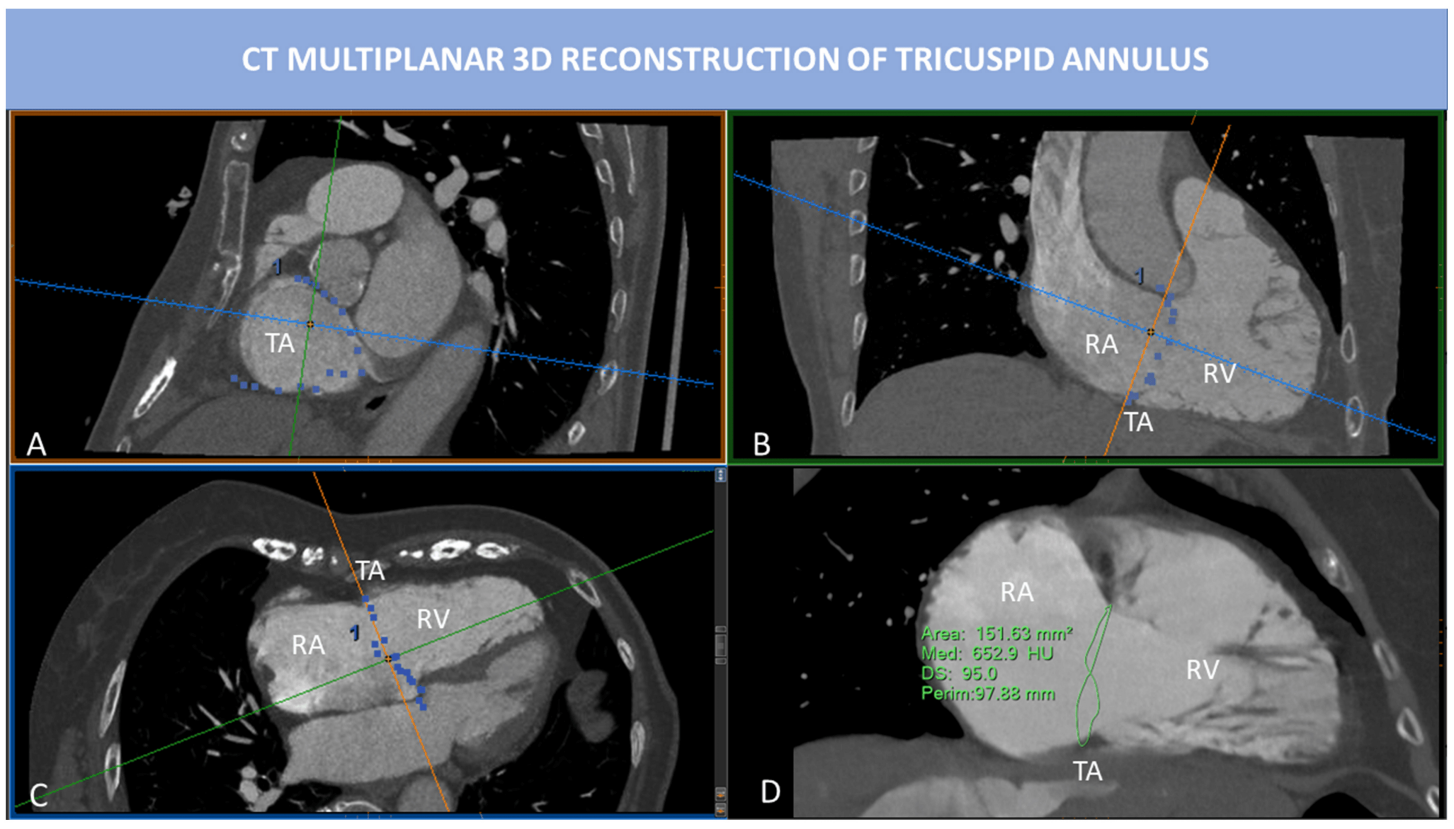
CT multiplanar 3D reconstruction of TA. MPR is used to
